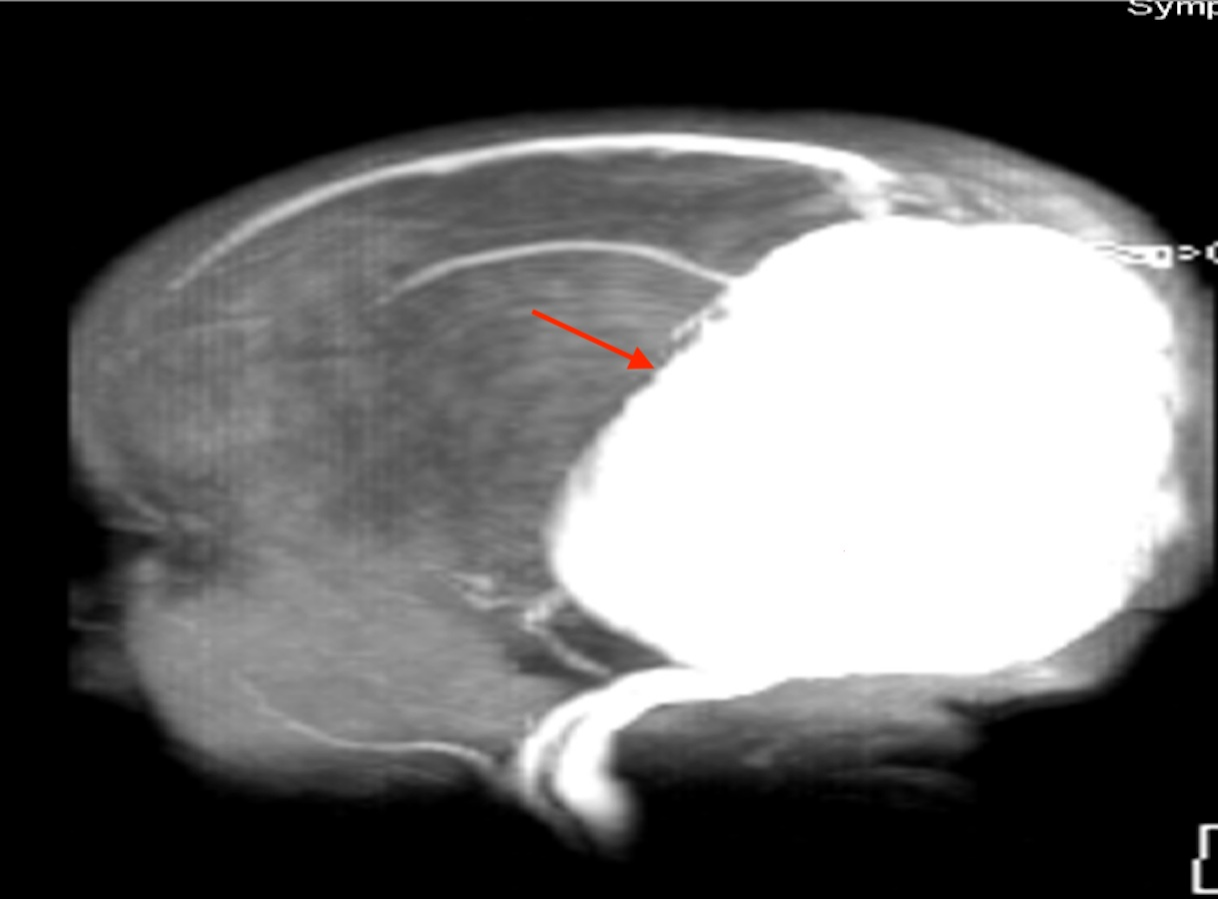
MRI scout image noting the large VGAM (red arrow) seen in the patient shown in Figure 2 MRI: magnetic resonance imaging; VGAM: vein of Galen aneurysmal malformation.
